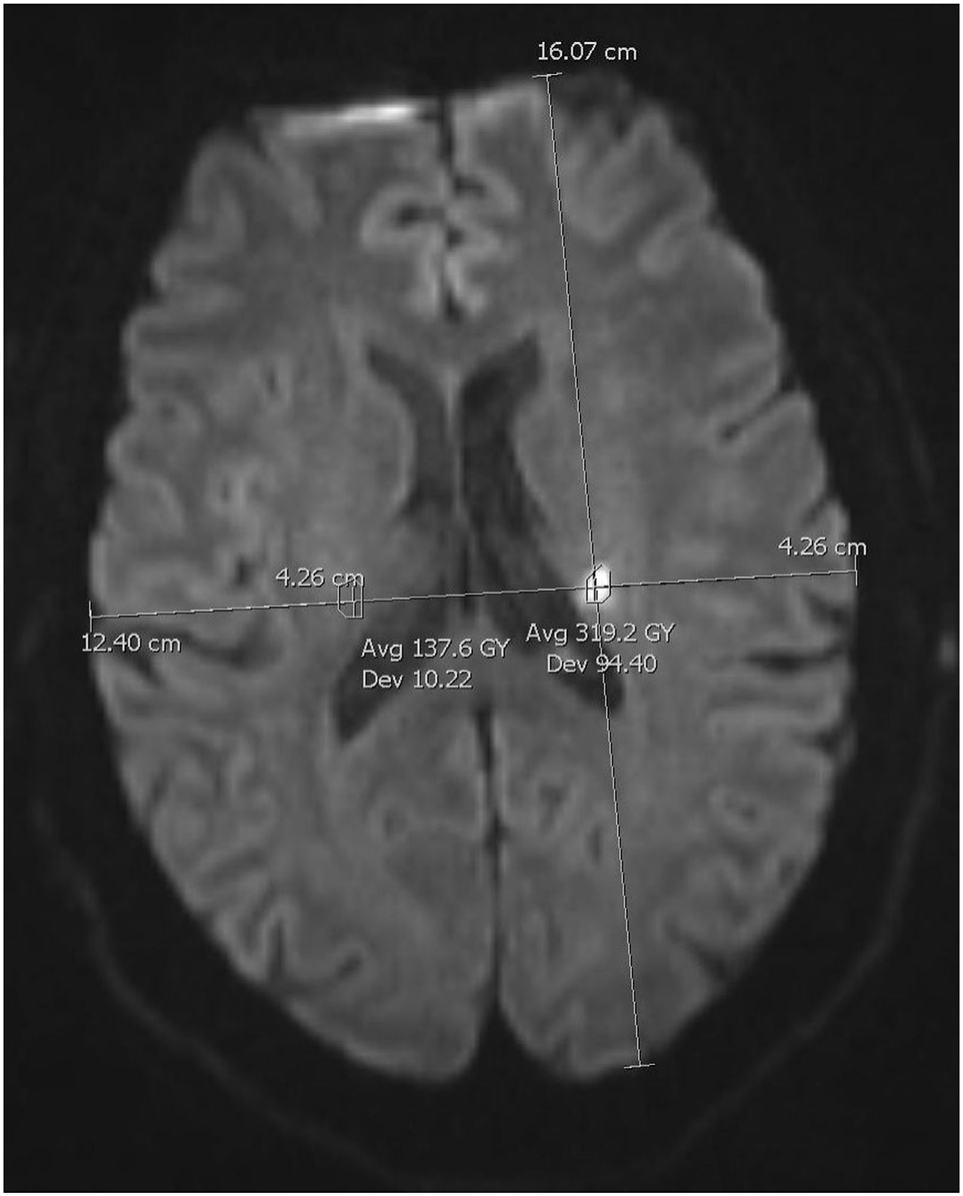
Regions of interest measurements. Co-registration method for lesion size and signal intensity on 3-gradient direction DWI.
